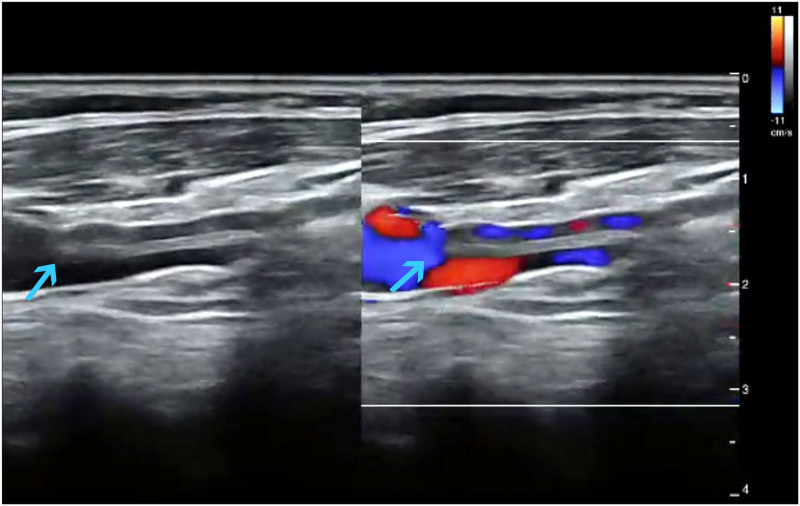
Ultrasound images of blood vessels in the neck revealed the internal jugular vein fenestration/duplication on a 5 to 8 MHz high-frequency probe (the arrows indicate the types of venous duplication).
